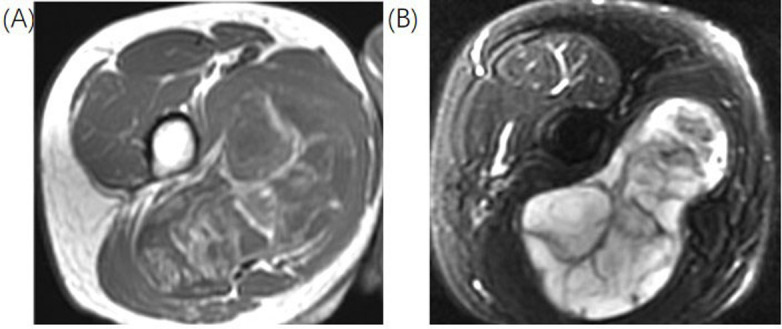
Representation of M-LPS. In this figure, there is the identification of a lesion in the medial compartment of the thigh. (A) Axial T2FS sequences with hyperintense of the lesion; (B) axial T1 sequences with hipointensity of the lesion. There are multiple internal settings of the lesion hypointense in the T2FS sequences and hyperintense in the T1 sequences. The lesion was proven at the biopsy a high-G M-LS. T2FS: T2-weighted fat-suppressed *Note.* Reprinted from Cancer Imaging Archive [Internet]. Frederick (MD): Frederick National Laboratory for Cancer Research. c2014–2020 [cited 2015 Jun 1]. Available from: https://wiki.cancerimagingarchive.net/pages/viewpage.action?pageId=21266533. CC BY.
